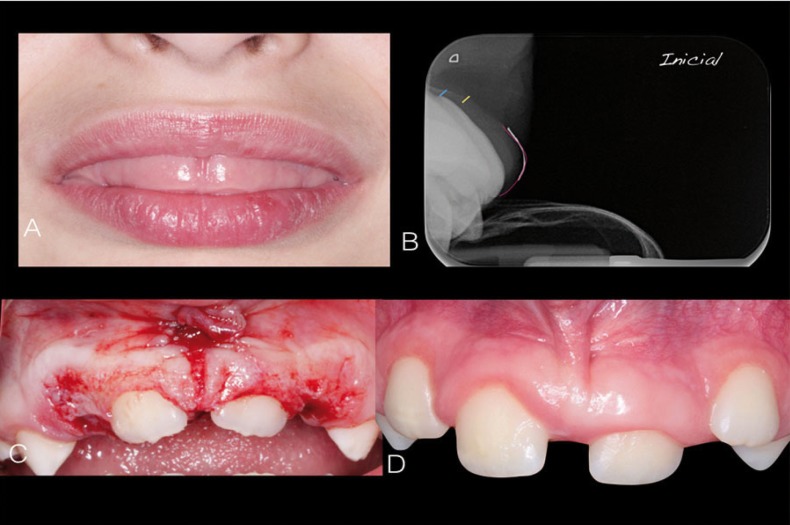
Case 2: A) Lack of lip closure; B) Parallel profile radiograph showing gingiva and bone crest relation; C) Intrasurgical view of external bevel incision gingivectomy; D) Intraoral view 24 months after surgery.
